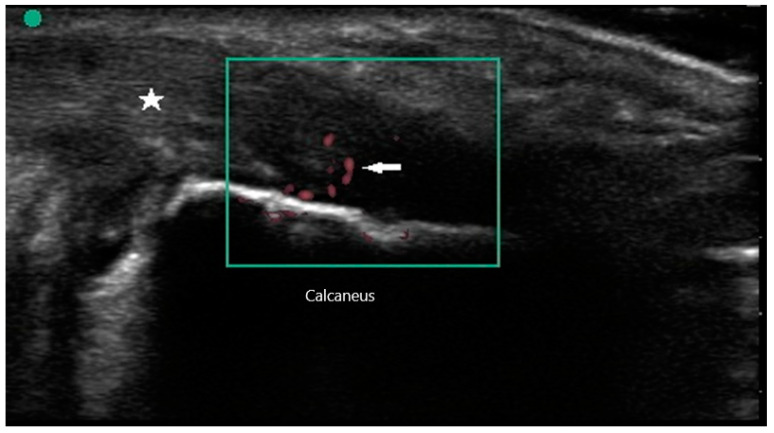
Enthesitis of Achilles tendon (star) with power Doppler signal (arrow).
